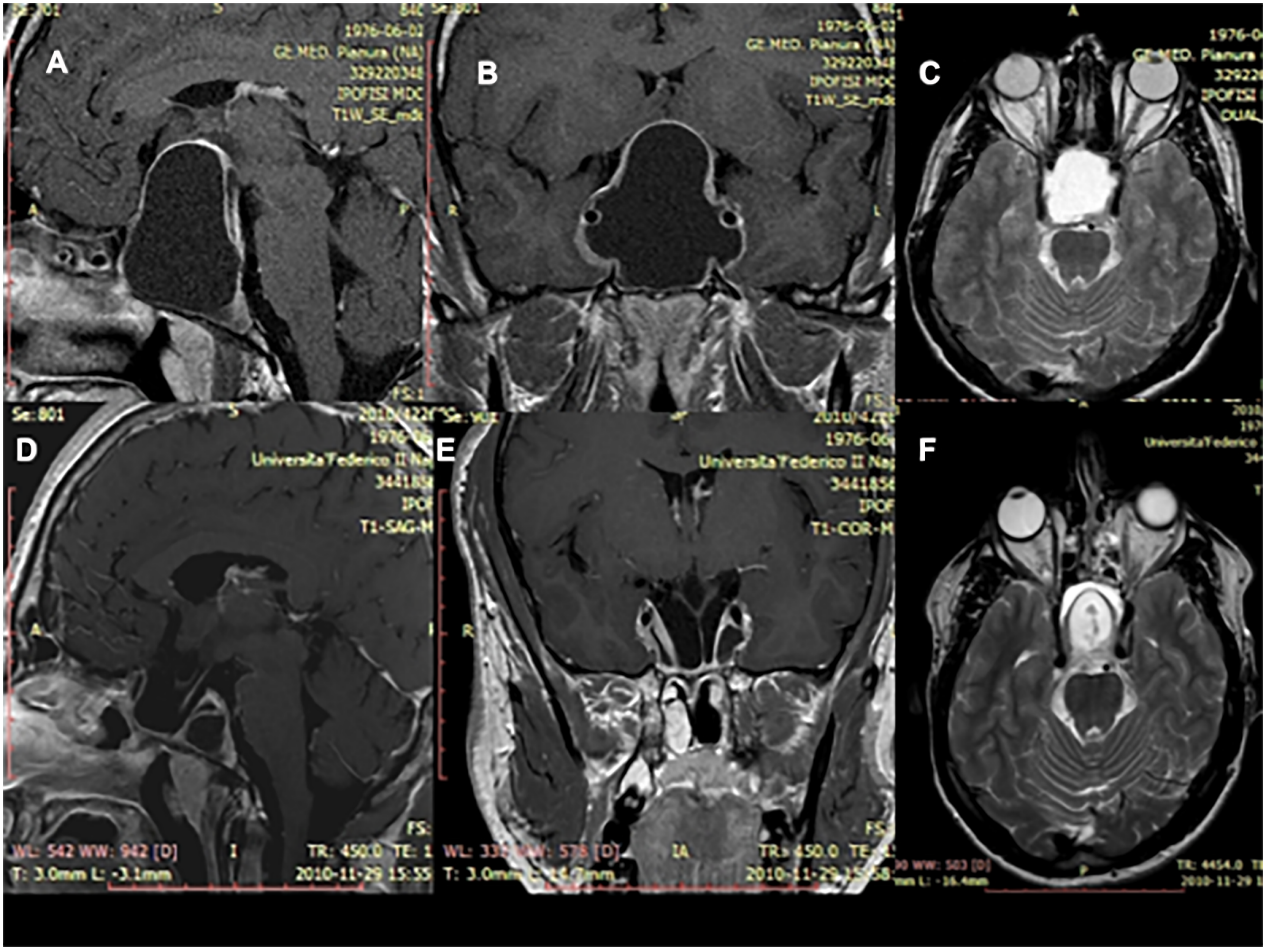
MRI scan after gadolinium showing an intra and suprasellar Rathke’s Cleft Cyst before and after the surgical removal via a standard endoscopic endonasal approach (case showed in the Fig 1). (A-B) Sagittal and a coronal T1-weighted scans of the lesion before being removed. The colloid has a hypointense signal and the cyst wall has post contrast enhancement. These features do not define typical aspect of RCC, whose differential diagnosis with sellar arachnoid cysts could be often challenging. (C) Axial T2-weighted scan of the lesion showing the colloid with a hyperintense signal. (D-E) Sagittal and a coronal T1-weighted scans and (F) axial T2-weightedscan at the three months postoperative MRI showing the cyst removal. It is possible to identify the decompression of the optic chiasm and the pituitary stalk.
